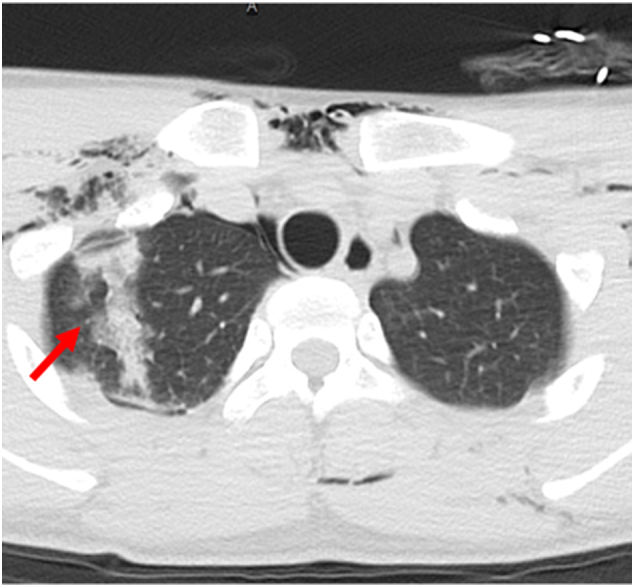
A chest computed tomography image taken by the previous doctor showing a pulmonary contusion at the apex of the lungs (red arrow). (For interpretation of the references to colour in this figure legend, the reader is referred to the web version of this article.)
